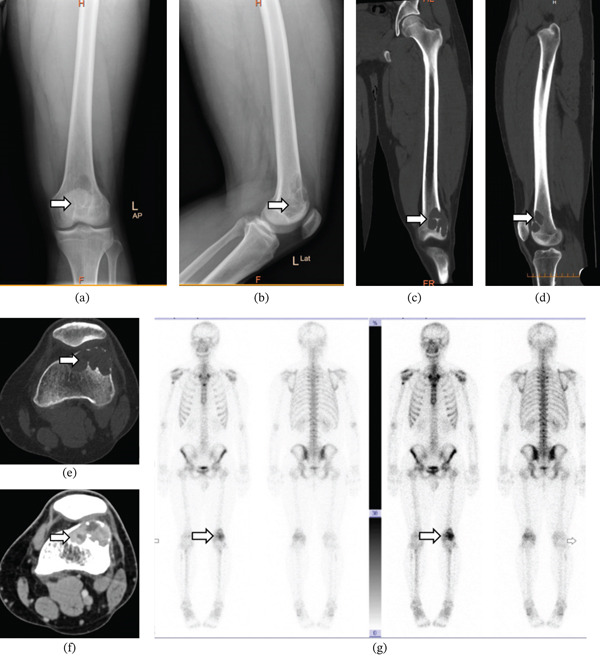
(a–b) Anteroposterior and lateral x‐ray examination of the left femur: Osteolytic bone destruction at the anterior margin of the lower segment. Notable findings include swelling, thinning, and discontinuity of the adjacent cortical bone, as well as roughness observed in the local cortical surface. (c–f) CT imaging of the lower left femur: An expansive, osteolytic area characterized by bone destruction accompanied by a soft tissue mass located at the anterior edge of the lower left femur. Within this region, there is a soft tissue density shadow present; however, no sclerotic margin is observed at the periphery. The boundary of the bone destruction area is distinctly defined, and there are no interruptions or discontinuities noted in the local bone cortex. (g) Whole body bone static imaging: Abnormal metabolic activity observed in the upper segment of the right humerus and at the distal end of the left femur.
